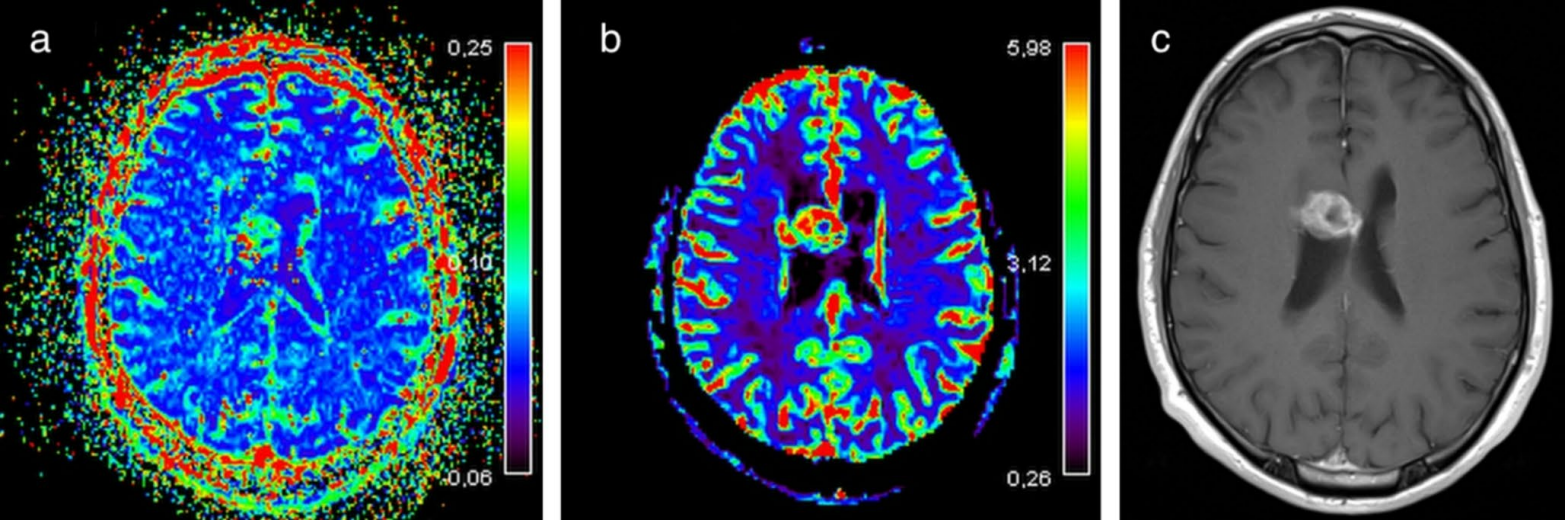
Recurrent tumor, neuropathologically confirmed ependymoma grade III. The f map (a) and rCBV map (b) show visual increase in their respective values in the lesion located close to the midline. The co-registered contrast-enhanced T1-weighted image (c) was overlaid on the perfusion maps when measurements were made
